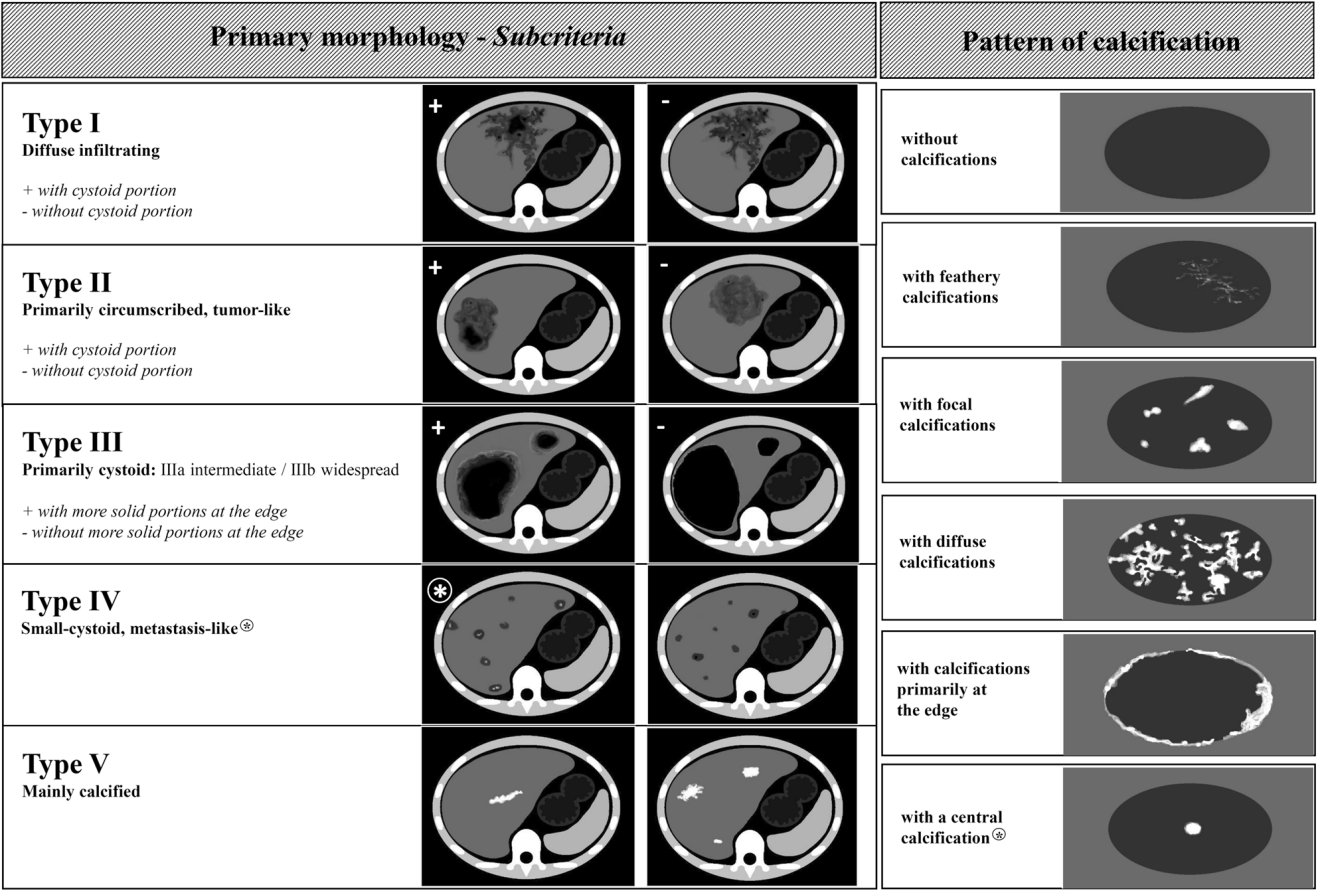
Overview of the EMUC-CT classification system, based on Graeter et al*. *(Left) Primary morphological types I–V and their subcriteria. (Right) Patterns of calcification. (EMUC-CT = *Echinococcus multilocularis* Ulm Classification for Computed Tomography). The two columns of classification are primarily considered separately; then, in principal, they can be freely combined. There are two exceptions: (1) The calcification pattern called “with a central calcification*” can only occur in lesions with type IV primary morphology, and (2) primary morphology type V is not associated with a pattern of calcification.
